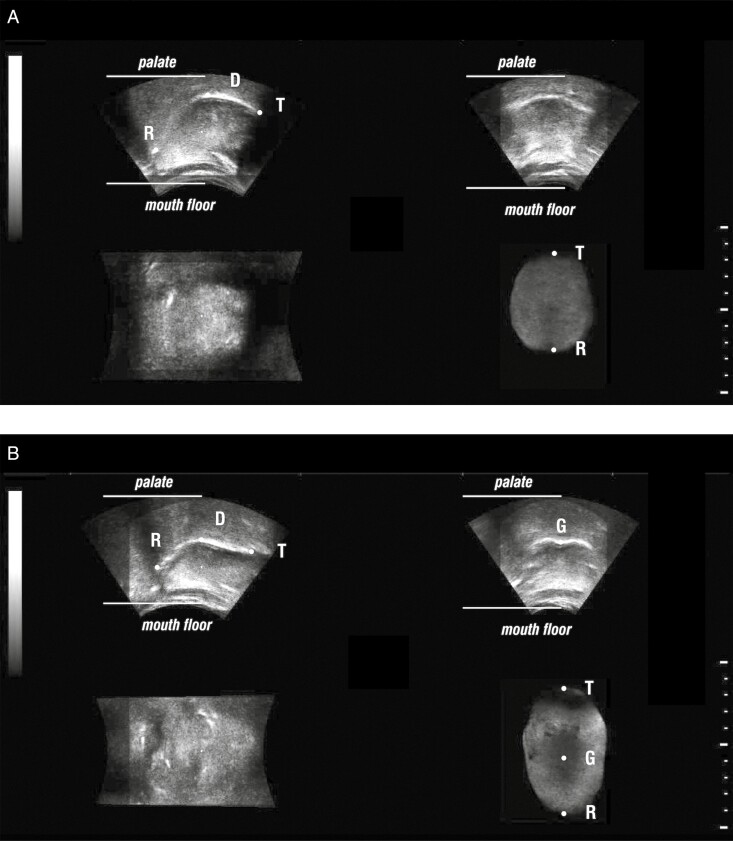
3-D ultrasonograms of tongue postured on the palate, with its convex surface and no groove in Fig. 3a, while the characteristic groove is visible in Fig. 3b, meaning that the tongue is postured on the mouth floor; R—tongue radix, D—tongue dorsum, T—tongue tip, G—groove.
